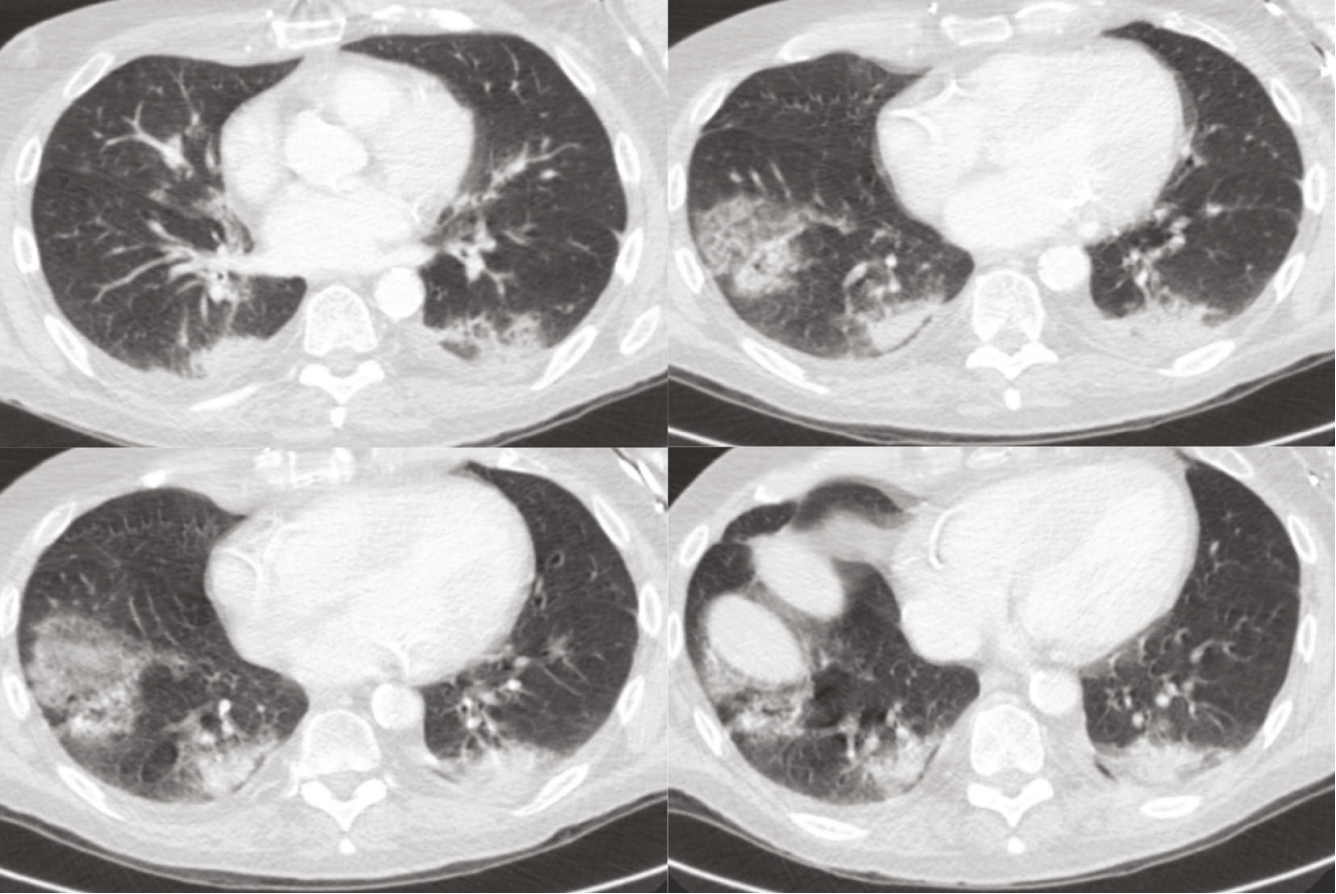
Computed tomography images of case #3.
